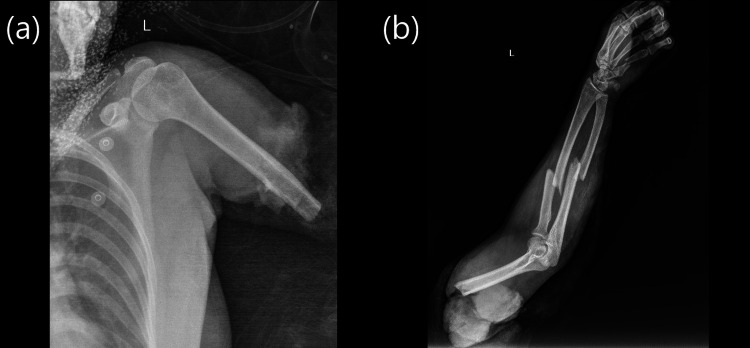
X-ray images of the patient’s left upper extremity (a, b).
